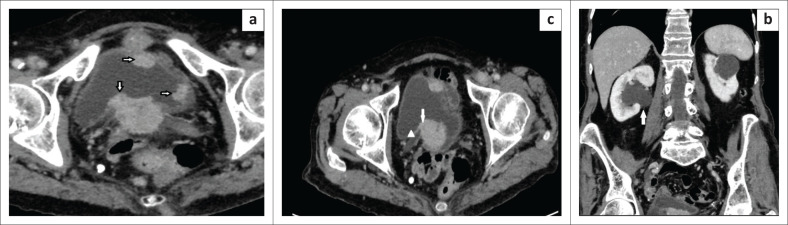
Abdomino-pelvic CT (a) multiple urinary bladder enhancing exophytic mural mass lesions (white arrows) (b) lesion (white arrow) located in the posterior bladder wall infiltrates the right vesico-ureteric junction (white arrow head), causing upstream obstructive uropathy (c) dilatation of the right renal pelvis (white arrow) secondary to tumour infiltrating the right vesico-ureteric junction.
